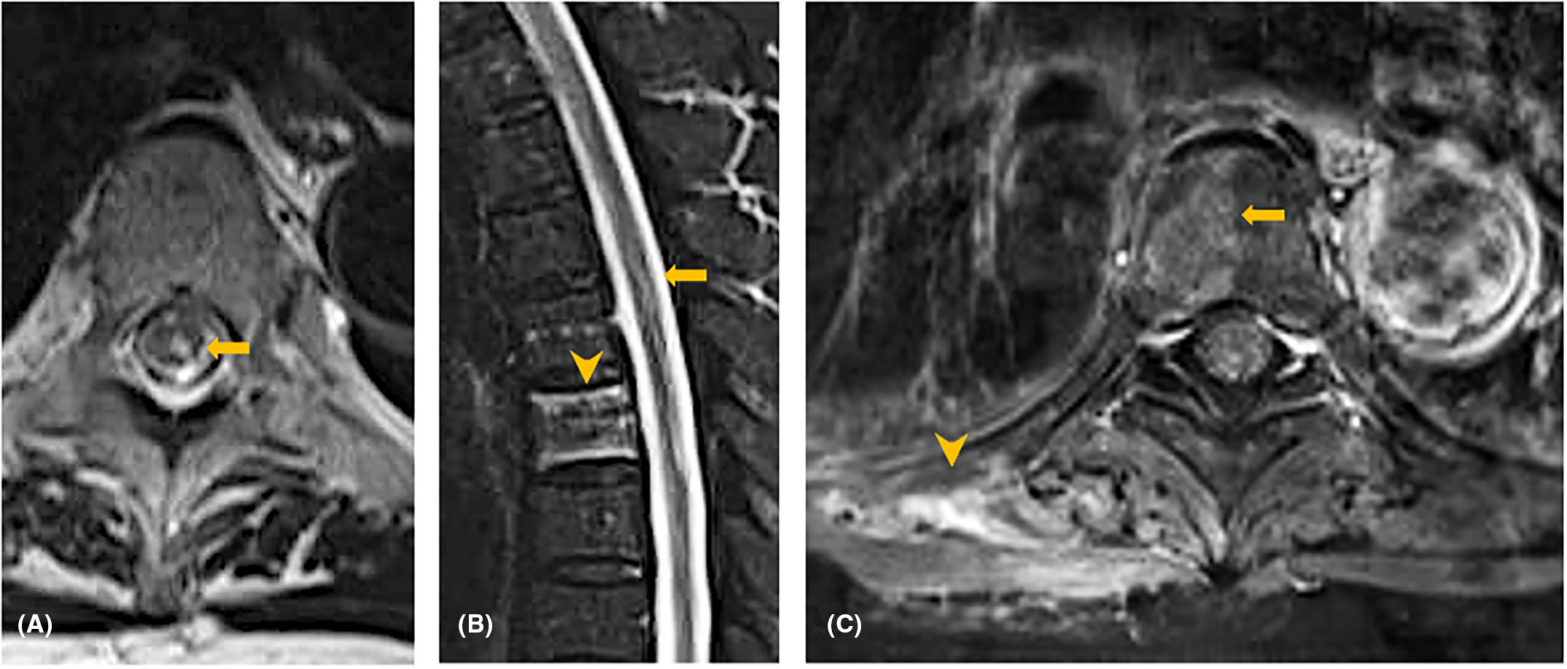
(A) T2‐weighted magnetic resonance imaging (MRI) revealed hyperintensity spot in the spinal cord at T5 level on axial view (arrow). (B) T2‐weighted MRI revealed longitudinal hyperintense lesion in the spinal cord at T5–T6 level (arrow), and hyperintense lesion in the T6 vertebral body (arrowhead) on sagittal view. (C) Gadolinium‐enhanced T1‐weighted MRI revealed high‐intensity areas in the right half of the T6 vertebral body (arrow) expanding to the right latissimus dorsi on axial view (arrowhead).
